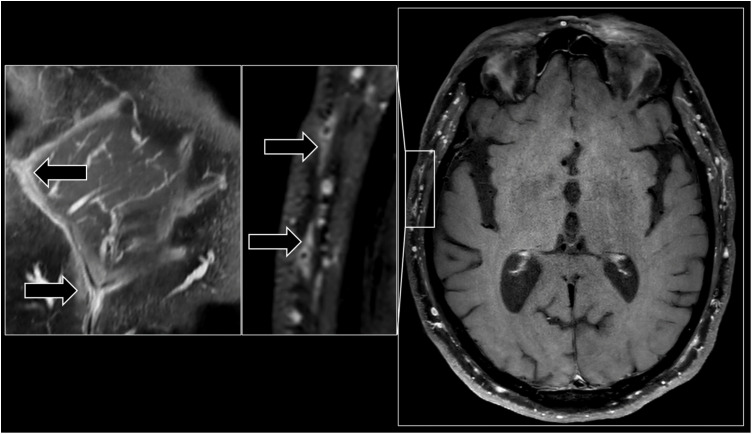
High-resolution vessel-wall MRI of temporal arteries in a patient with biopsy-proven giant cell arteritis. Seventy-two-year-old man with a positive diagnosis of GCA on post-contrast three-dimensional fat-saturated turbo spin echo high resolution vessel-wall imaging dedicated for visualizing the extracranial artery wall. Multiplanar reconstruction (left images) and axial plane (right image) showing a marked wall thickening (> 0.7 mm) and strong mural enhancement with perivascular inflammatory infiltration of temporal arteries (arrows).
